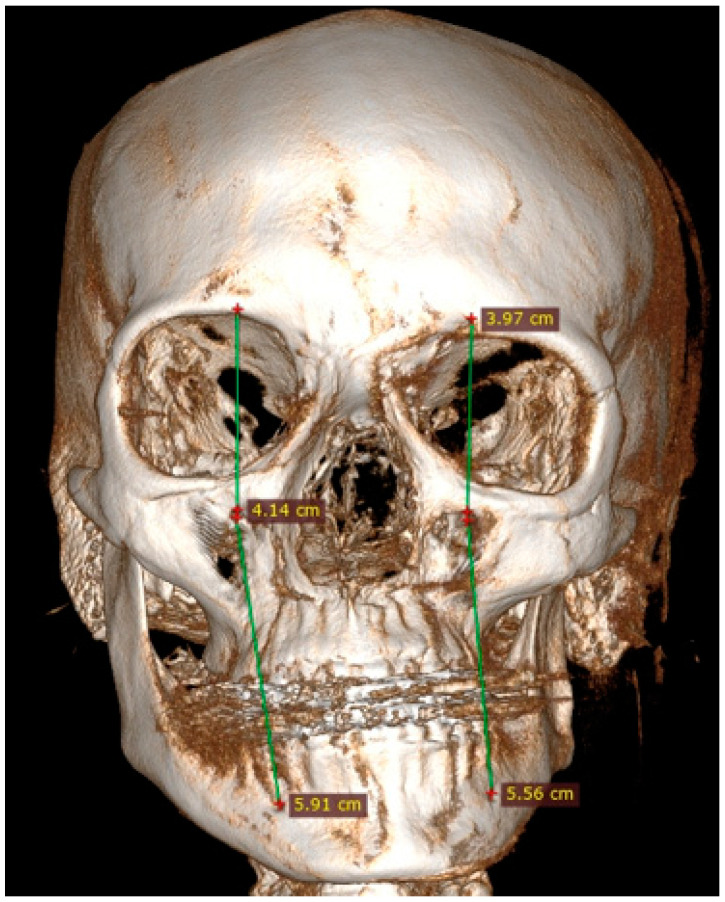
CBCT measurements in maxillo-mandibular asymmetry caused by right-sided condylar hyperplasia—the measurements in vertical aspect: SOF-IOF and IOF-MFO. Vertical lines are useful to indicate the disturbances in symmetry.
